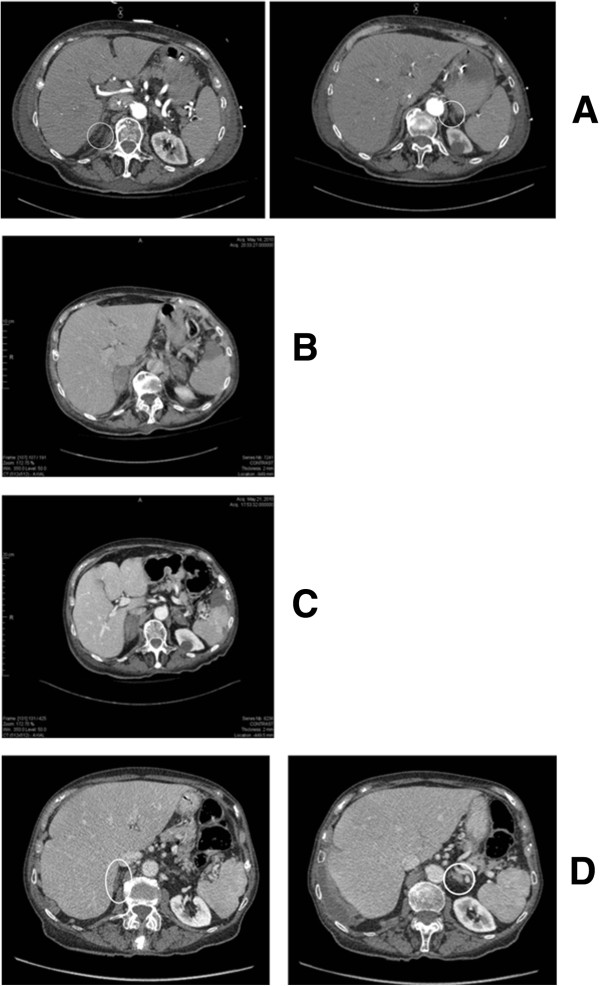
Abdominal computed tomography scans. (A) Abdominal computed tomography scan on day 3 showing normal adrenal glands. (B) Abdominal computed tomography scan on day 14 showing infiltration with increased adrenal gland volume. (C) Abdominal computed tomography scan on day 21 showing bilateral adrenal hemorrhagic infarction. (D) Abdominal computed tomography scan on day 435 showing small atrophic adrenal glands.
